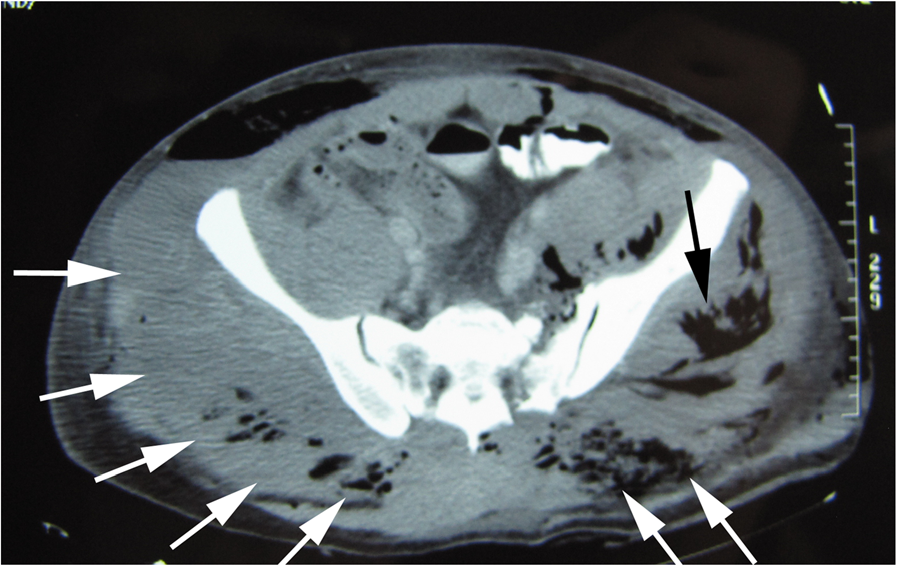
Computed tomography seven days after injury showed left gluteal muscle damage (black arrow), and a massive bilateral collection of fluid and gas between the gluteal muscles and subcutaneous soft tissue (white arrows).
